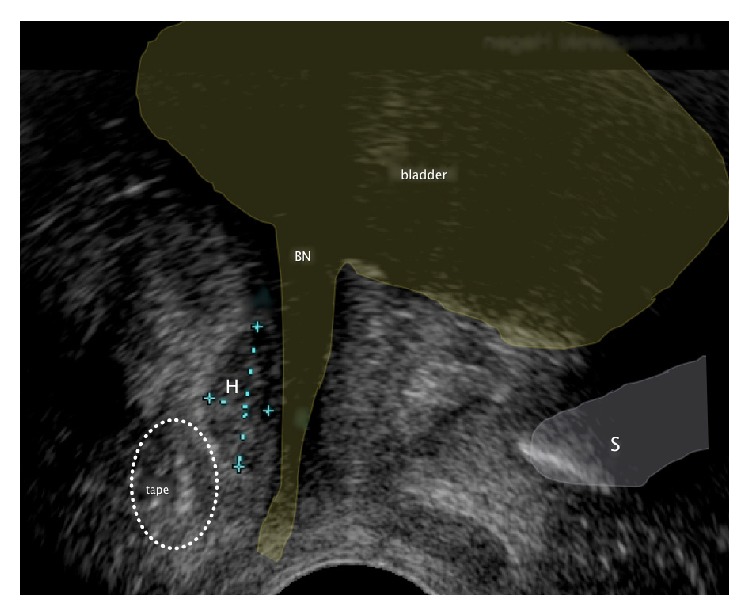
Pelvic floor ultrasound examination in sagittal plane. A small hematoma between TVT and the urethra leads to compression of the urethra and to transient voiding problems. No operative intervention is required. S: symphysis pubis, BN: bladder neck, and H: hematoma.
